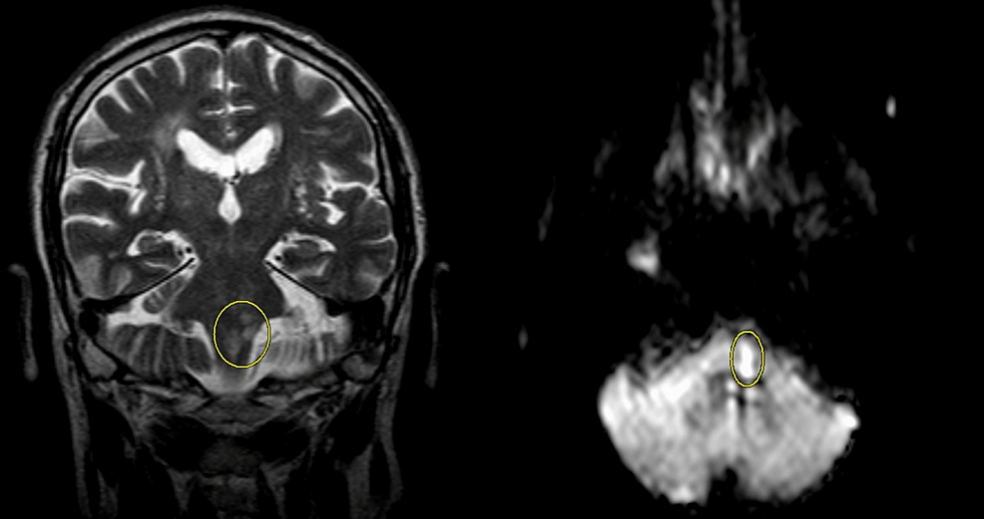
MRI of the brain without contrast showing restricted diffusion
